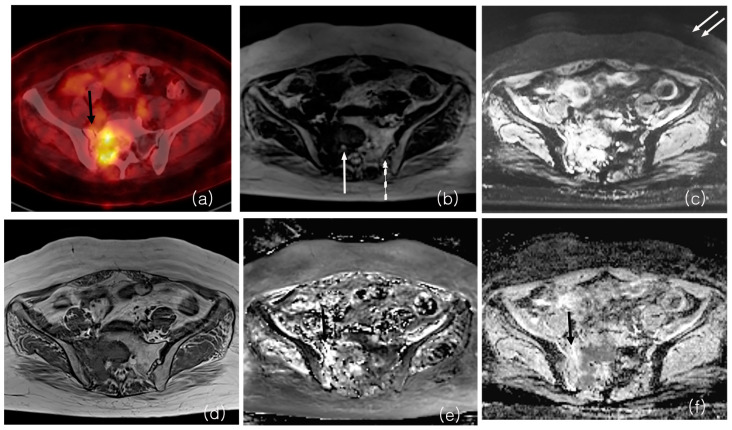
MRI and 18F-FDG PET/CT from a patient with bone metastasis from breast cancer. PET/CT shows a sacral lesion with increased FDG uptake, with SUV peak measuring 7.21 (a). BLADE b value 800 DWI (b) and ADC map (e) shows the lesion in the sacral body and right ala (ADC max 2924). RESOLVE b value 800 DWI (c) and ADC map (f) also shows the lesion (ADC max 2632). There is a fracture line in the right sacral ala, with less intense FDG uptake on PET and higher ADC value on MRI (black arrows, (a,e,f)). On image quality analysis, anatomical details such as neural foramen (white arrow) and sacroiliac joint (dotted arrow) are better visualized on BLADE (b) and less distinct on RESOLVE b value 800 DWI (c). Artifact in background air is more prominent on RESOLVE (double arrows on (c)). T1-weighted non-contrast MRI (d) is shown as a reference image.
